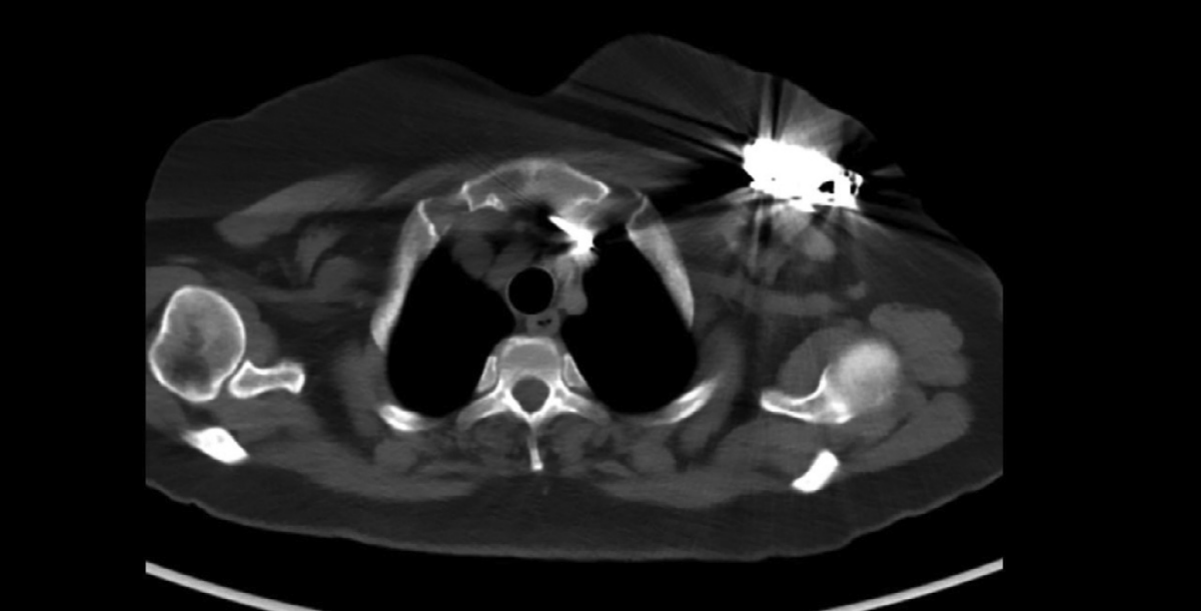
CT scans axial view show metal artifact in the left infra-clavicular region corresponding to a cardiac ICD.
